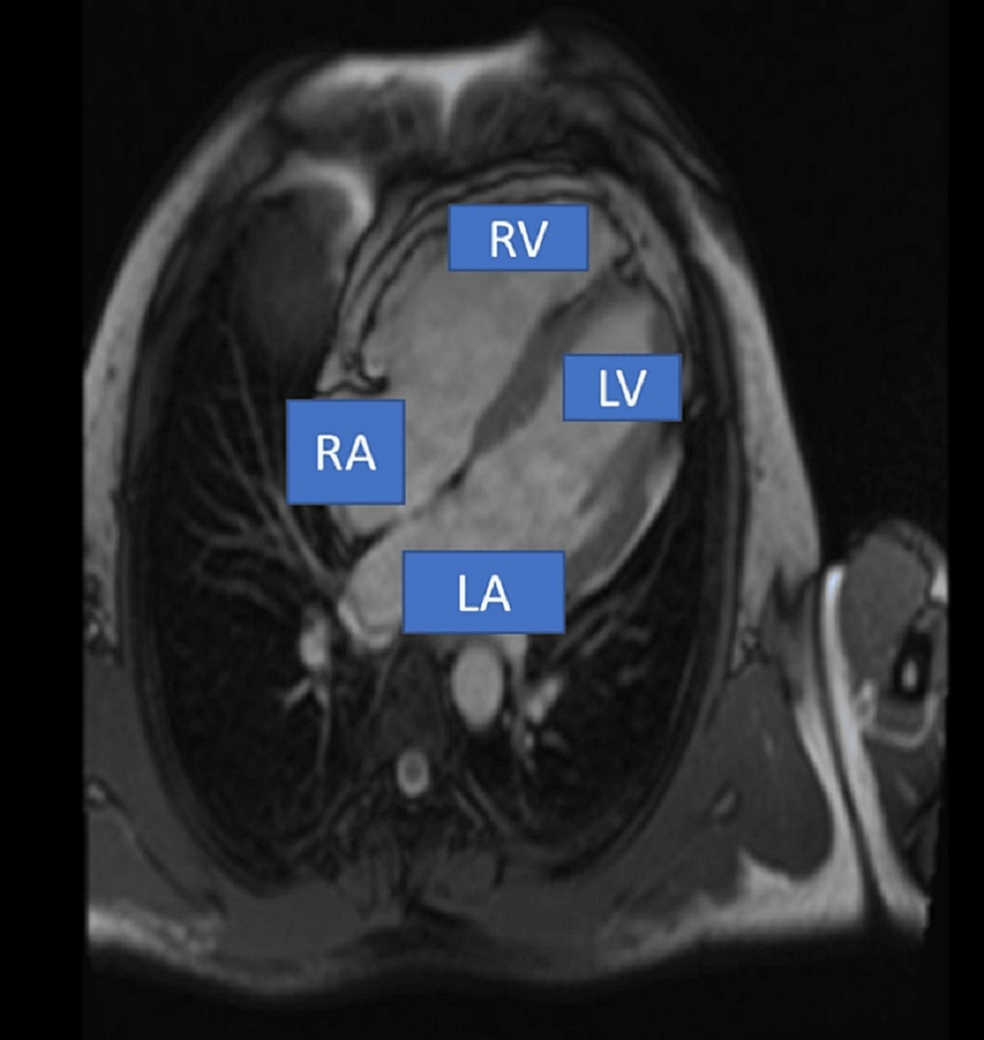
MRI showed grossly deranged right ventricular function with outpouching and thinned out apical segment with late gadolinium enhancement of right ventricular free wall. RA, right atrium; RV, right ventricle; LA, left atrium; LV, left ventricle
